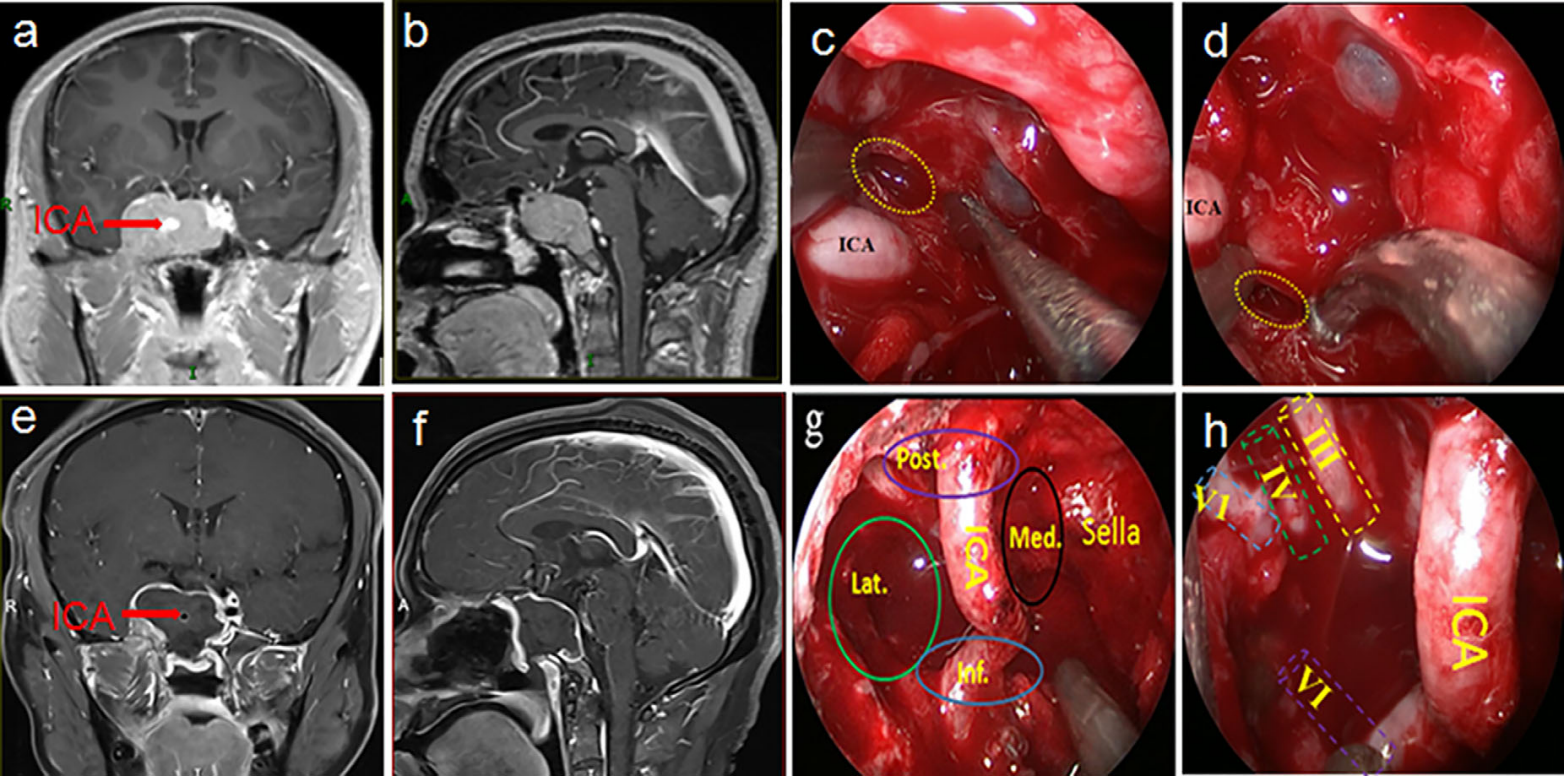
This was a 32-year-old male patient who was admitted to hospital after the accidental discovery of a pituitary tumor due to trauma. The preoperative visual examination was normal, and the preoperative PRL and ACTH hormones were increased. A preoperative MRI (A, B) showed that the tumor was in Knosp grade 4 and was mainly distributed in both posterosuperior and anteroinferior compartments of the CS. Intraoperatively, various compartments (G) and nerves (H) in the CS could be observed after total tumor resection. Postoperative MRI (E, F) also confirmed total tumor resection. The yellow dotted circle indicated entry into the CS from above (C) and below (D) the horizontal ICA. The pathological examination of the tumor was mixed pituitary adenoma. The visual and endocrine function of the patient were all normal three months after the operation. Post., posterior compartment; Med., medial compartment; Lat., lateral compartment; Inf., inferior compartment; ICA, internal carotid artery; III, oculomotor nerve; IV, trochlear nerve; V1, first branch of the trigeminal nerve; VI, abducens nerve.
